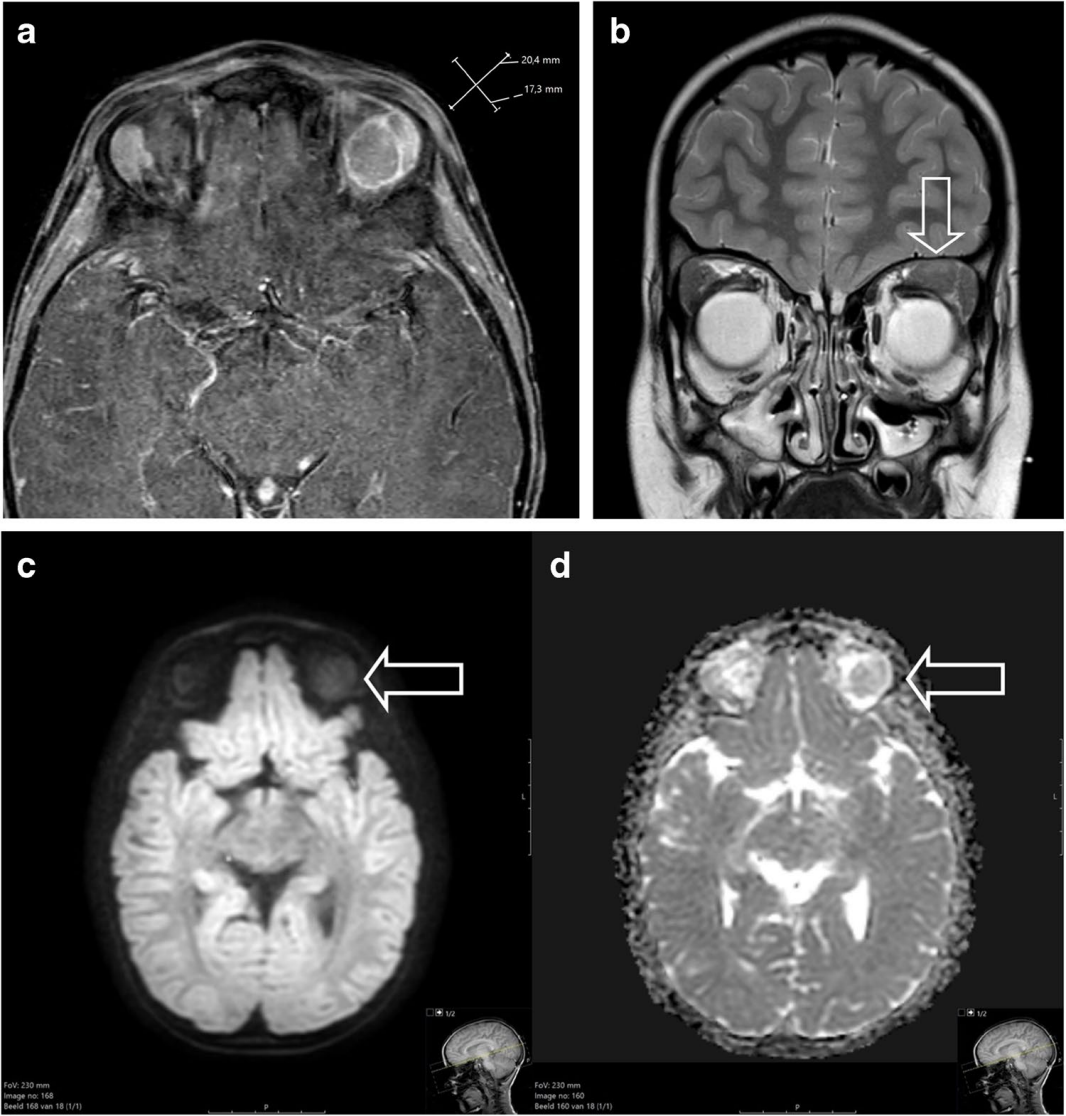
Response assessment magnetic resonance imaging (MRI) of a primary orbital rhabdomyosarcoma in a 6-year-old boy (patient 1) after three cycles of chemotherapy (comparison is made with the baseline MRI study . See also Fig. 3). a Axial T1 image after gadolinium administration with fat suppression shows partial remission of the tumour (30–100% volume response) after three months of chemotherapy. b Coronal T2 turbo spin echo image shows decreased signal intensity and diminished compression on the globe (*arrow*). The axial diffusion-weighted (b-value 800) (c) and axial apparent diffusion coefficient mapping (d) images show less restricted diffusion (*arrows*)
